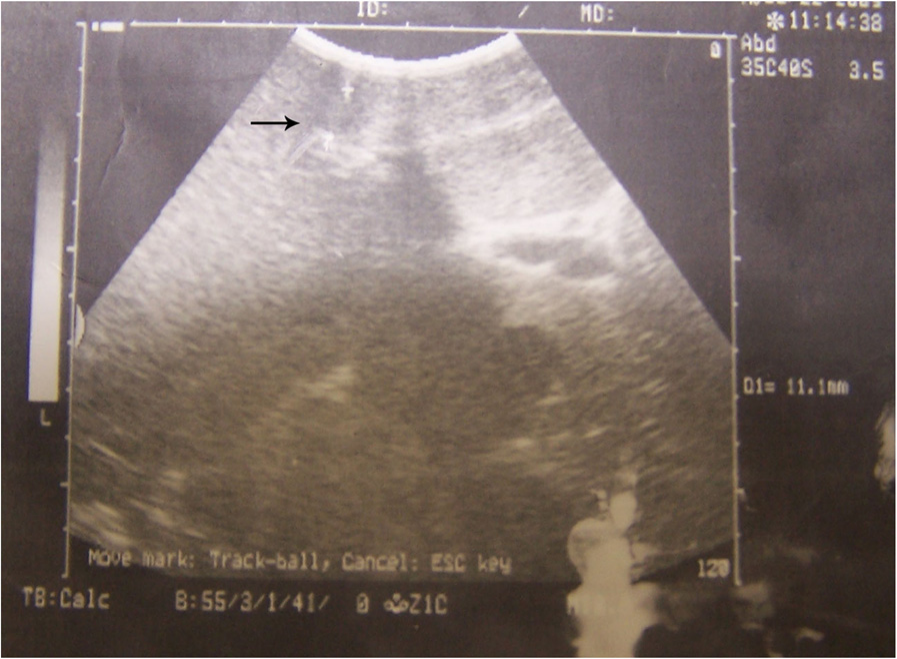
Intraoperative findings. Intraoperative ultrasonography. The arrow indicates the location of the pseudolesion.
